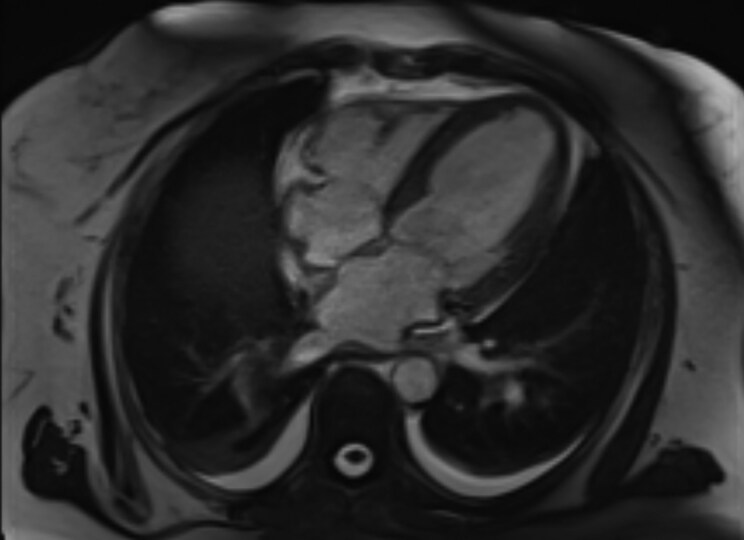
Four-chamber CINE cardiac MRI demonstrating myocardial oedema and inflammation in the acute phase. Findings include increased T2 signal in affected segments consistent with acute myocardial oedema.
